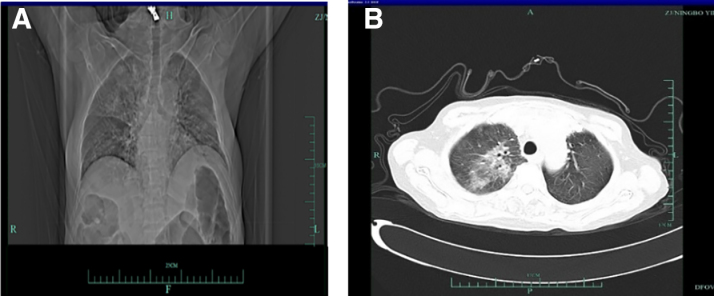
Chest CT image obtained on January 27, 2018, during the patient’s final admission. Compared with previous studies, this scan shows progressive worsening of bilateral pulmonary infectious infiltrates with interstitial changes and a newly developed bulla in the left lower lobe (indicated by the red arrow in the figure) (A, B). The anterior mediastinal region exhibits residual soft tissue opacity. These findings represent end-stage pulmonary damage resulting from recurrent infections in the setting of severe combined immunodeficiency. CT = computed tomography.
